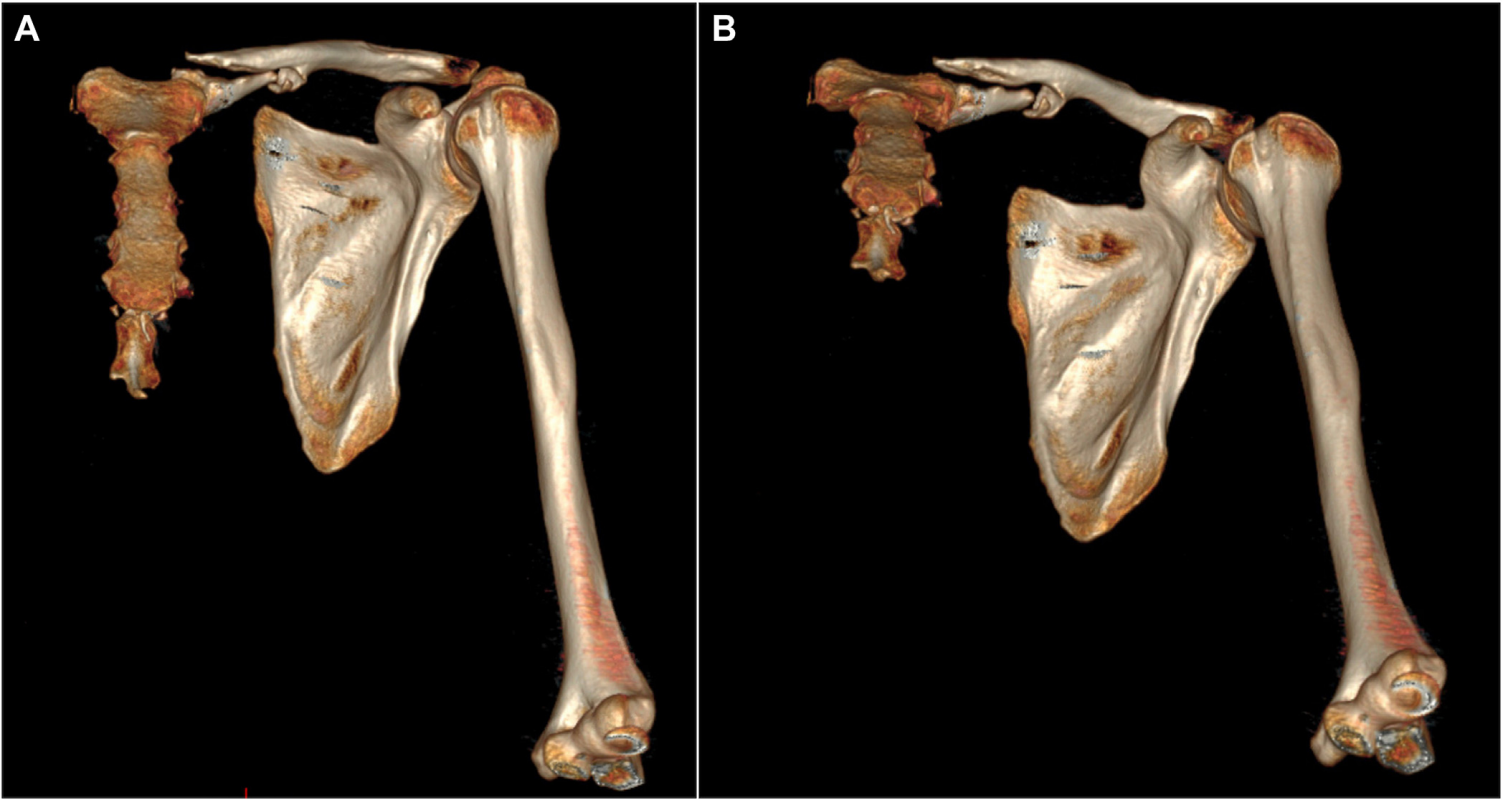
Preoperative three-dimensional reconstructions from computed tomographic imaging show a nonunion of the medial clavicle, obtained at 11 months after initial injury. Two perspectives are shown, including anteroposterior view (A) and anteroposterior with cephalic angulation (B). There is a short segment of medial bone, which remains reduced to the sternum at the sternoclavicular joint, and an oblique fracture pattern.
